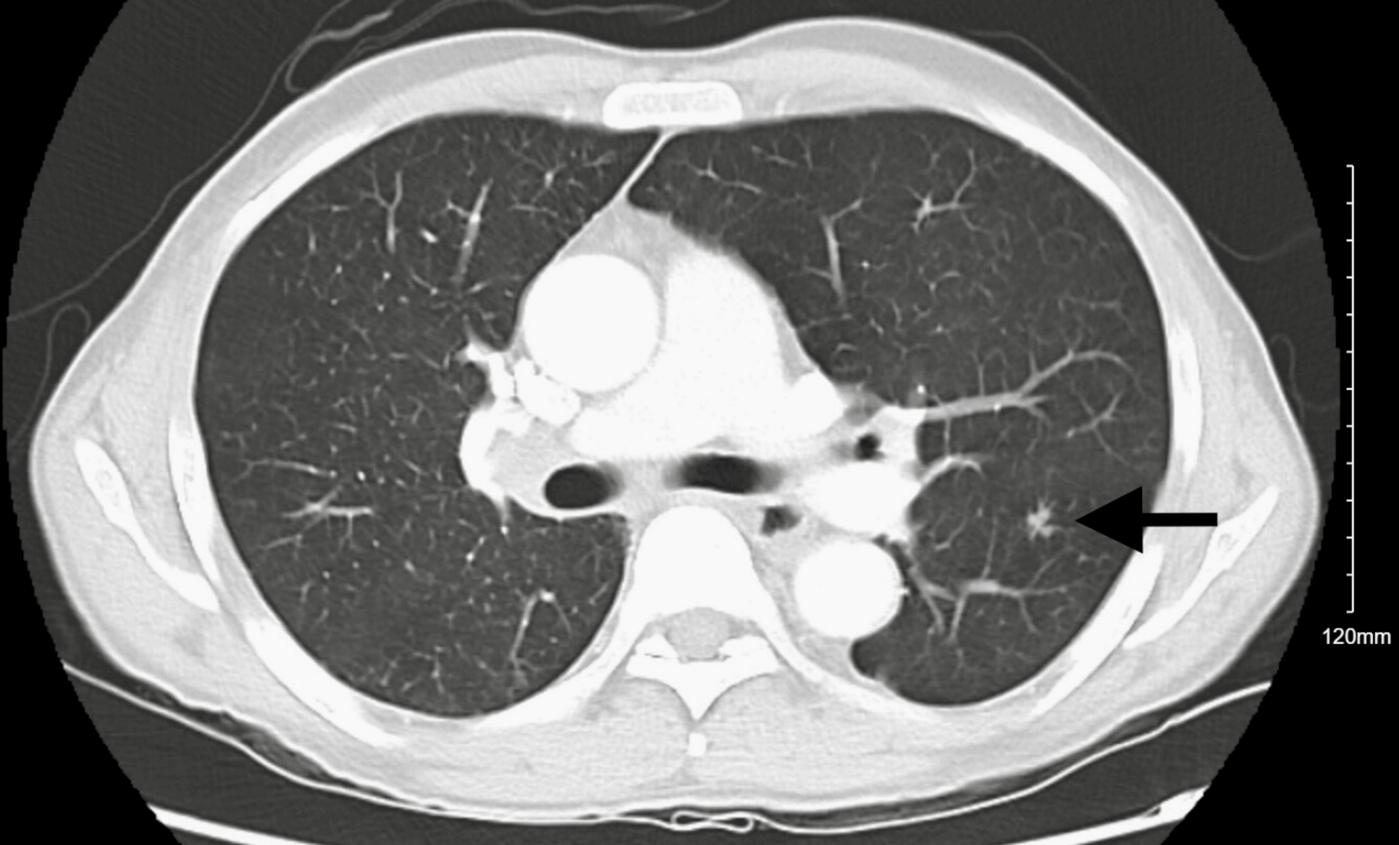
CT chest revealing tree-in-bud appearance
